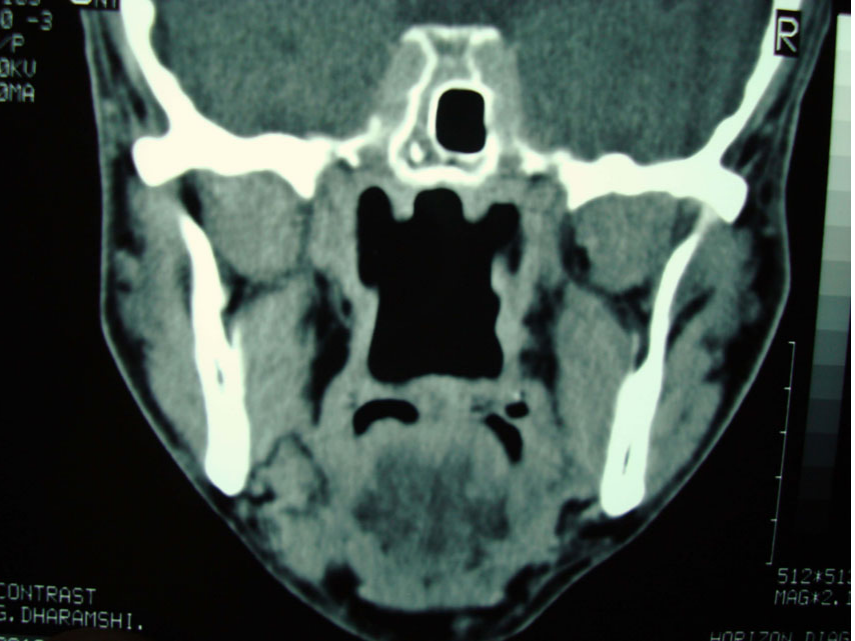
Post contrast CT of the same patient.
